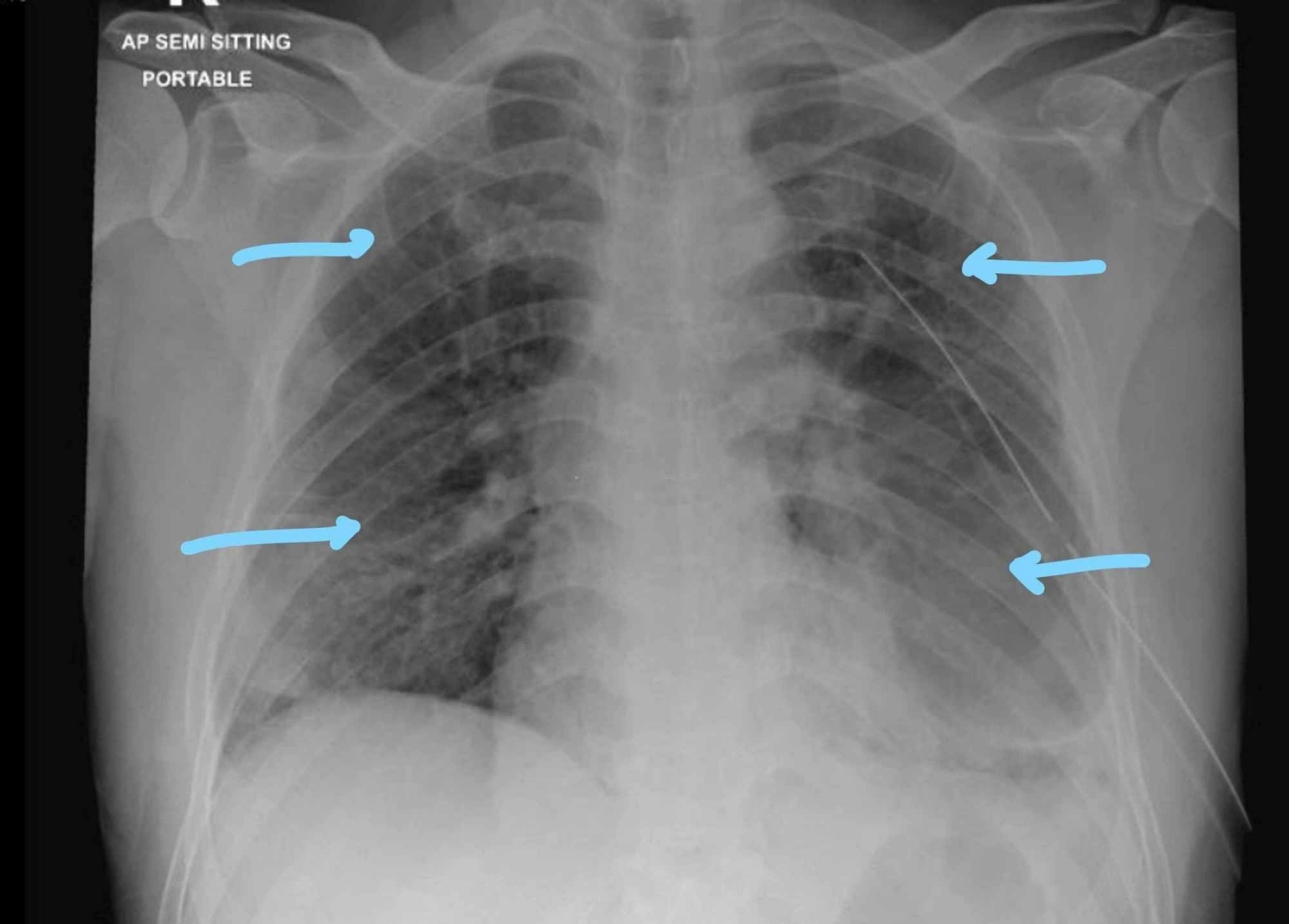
Repeat chest X-ray showing clamped chest tubes and inflated lungs
